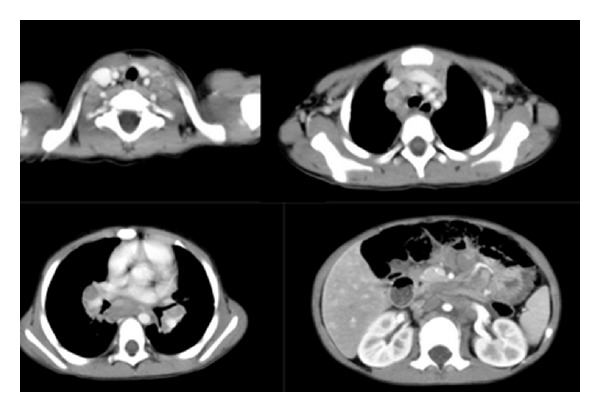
Thoracic and abdominal CT shows multiple lymph node enlargements in the regions of the bilateral neck and hilum, as well as the mediastinum and retroperitoneal areas.
